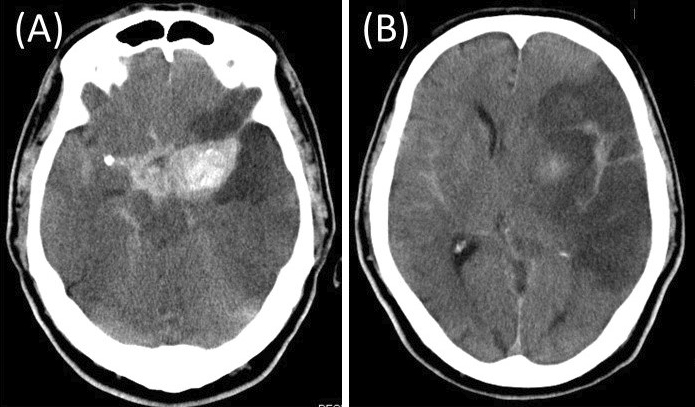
Computed tomography indicating a subarachnoid hemorrhage (A) and cerebral infarction in the territory of the MCA (B).
